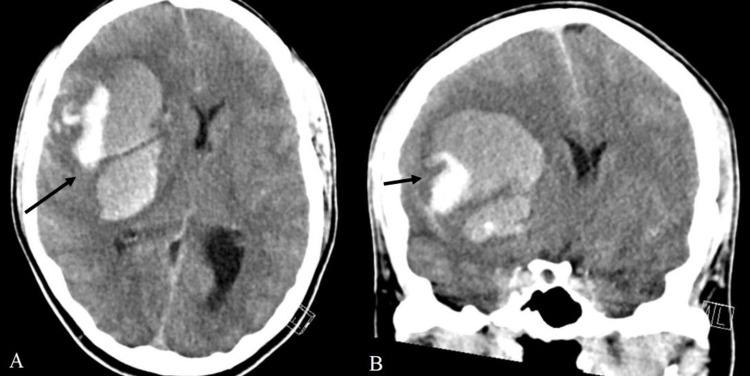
Head CT scan performed on presentation at the emergency department showing a large intraparenchymal hemorrhage in the right hemisphere. (A) Axial plane and (B) coronal plane.
